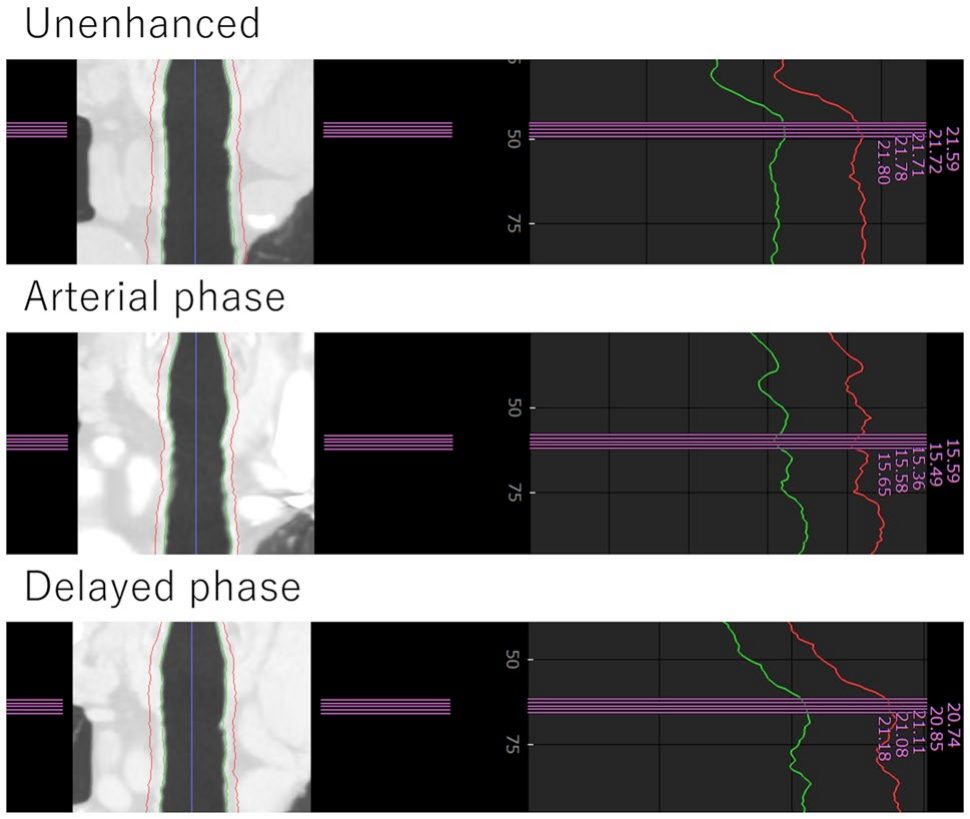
Representative CT images (left) and tracheal diameter data images (right) of an 85-year-old male patient in whom the difference of the tracheal diameter between unenhanced (21.7 mm) and arterial phase (15.4 mm) was the largest. There was no considerable misregistration for the trachea
